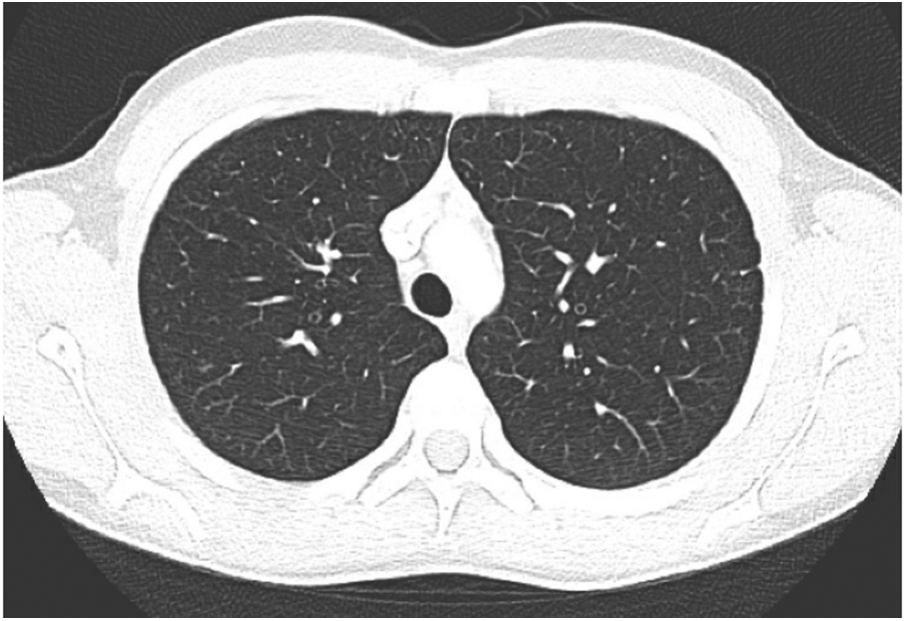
CT scan demonstrating interval near resolution of previously described punctate nodules throughout both lungs. Two isolated ground glass foci remain with the right lung. No new nodule or ground glass opacity.
